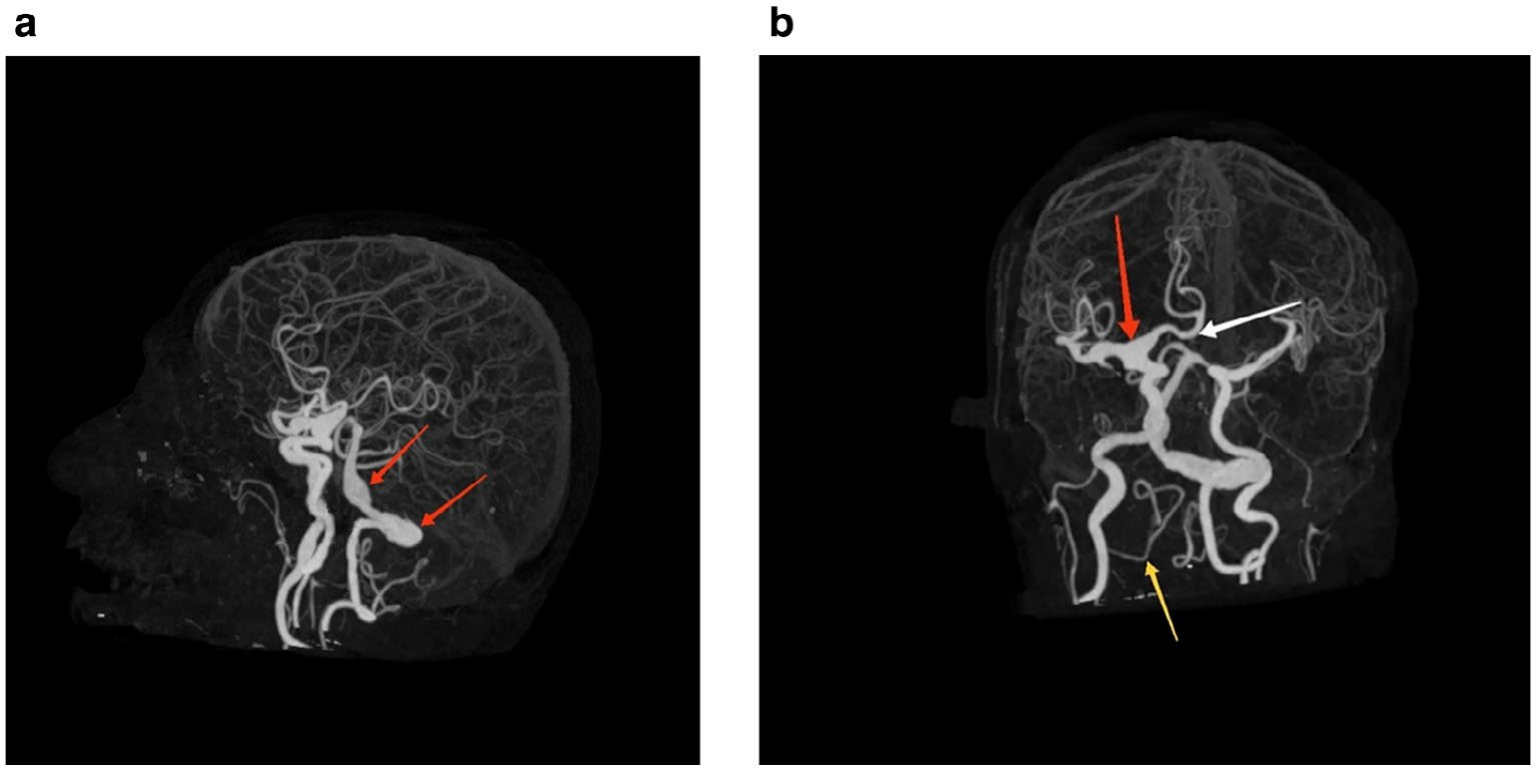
(a) CT cerebral angiography 3D image showing ectatic and torturous left vertebrobasilar artery (red arrow). (b) CT cerebral angiography 3D image showing ectatic terminal segment of internal carotid, proximal portion of M1 segment of right MCA and proximal portion of A1 segment of right ACA (red arrow). Absent left A1 segment of left ACA is noted with both ACA arising from A1 segment of right ACA (white arrow). Hypoplastic right vertebral artery is noted (yellow arrow). Left posterior inferior cerebellar artery is noted arising from left vertebral artery.
